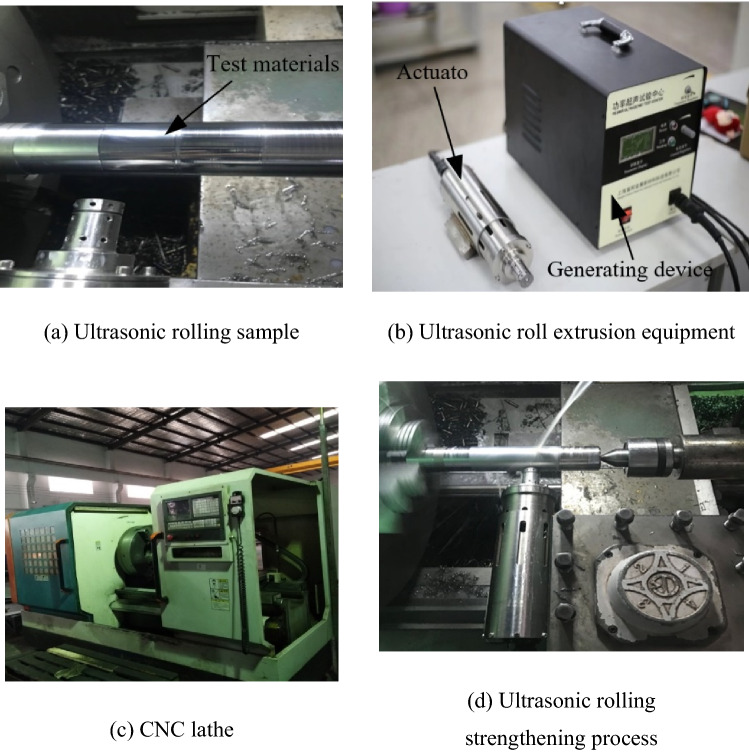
Ultrasonic roll extrusion.
